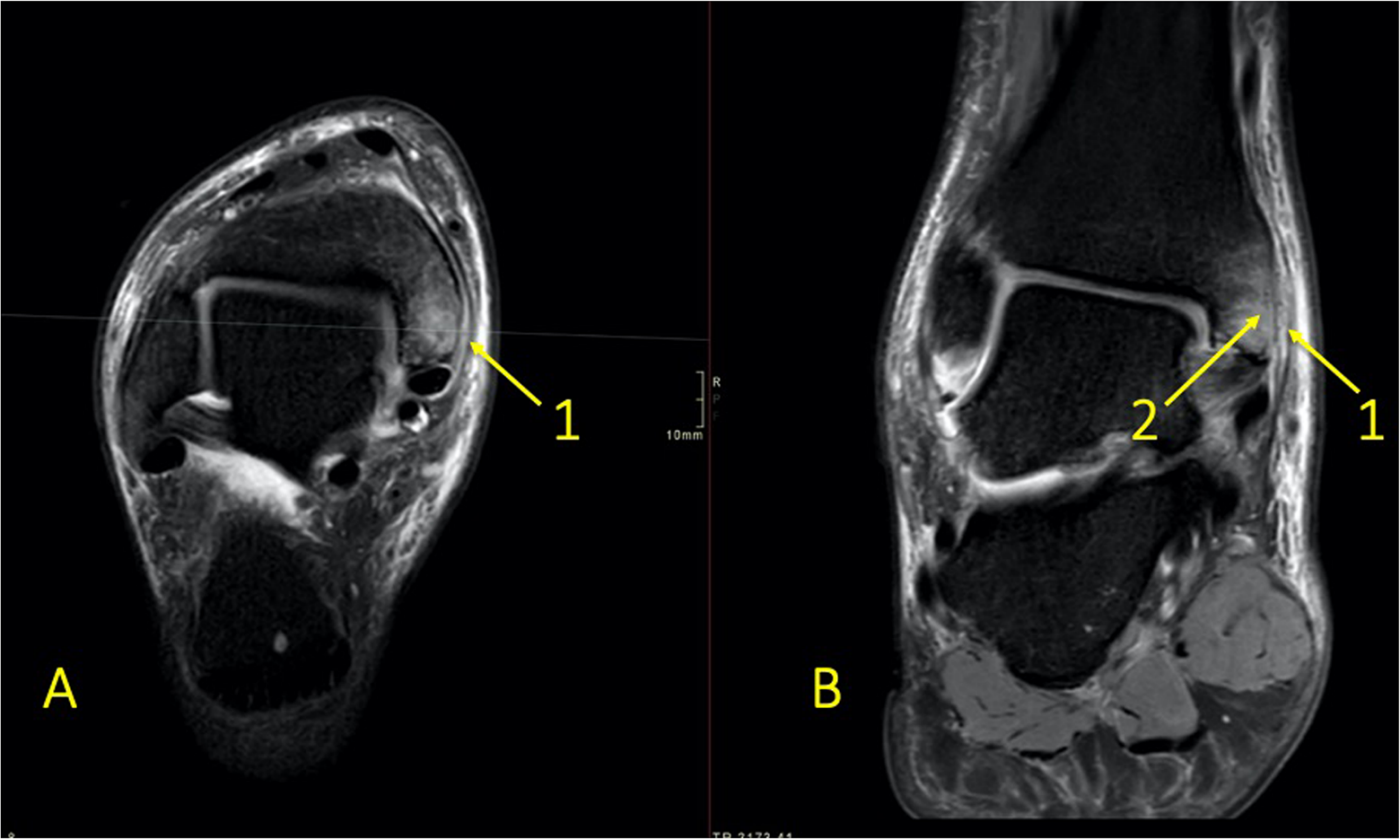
A 39-year-old male imaged 10 days after an ankle injury on suspicion of deltoid ligament rupture. MRI showed (1) partial injury of the flexor retinaculum with (2) BME in the medial malleolus
